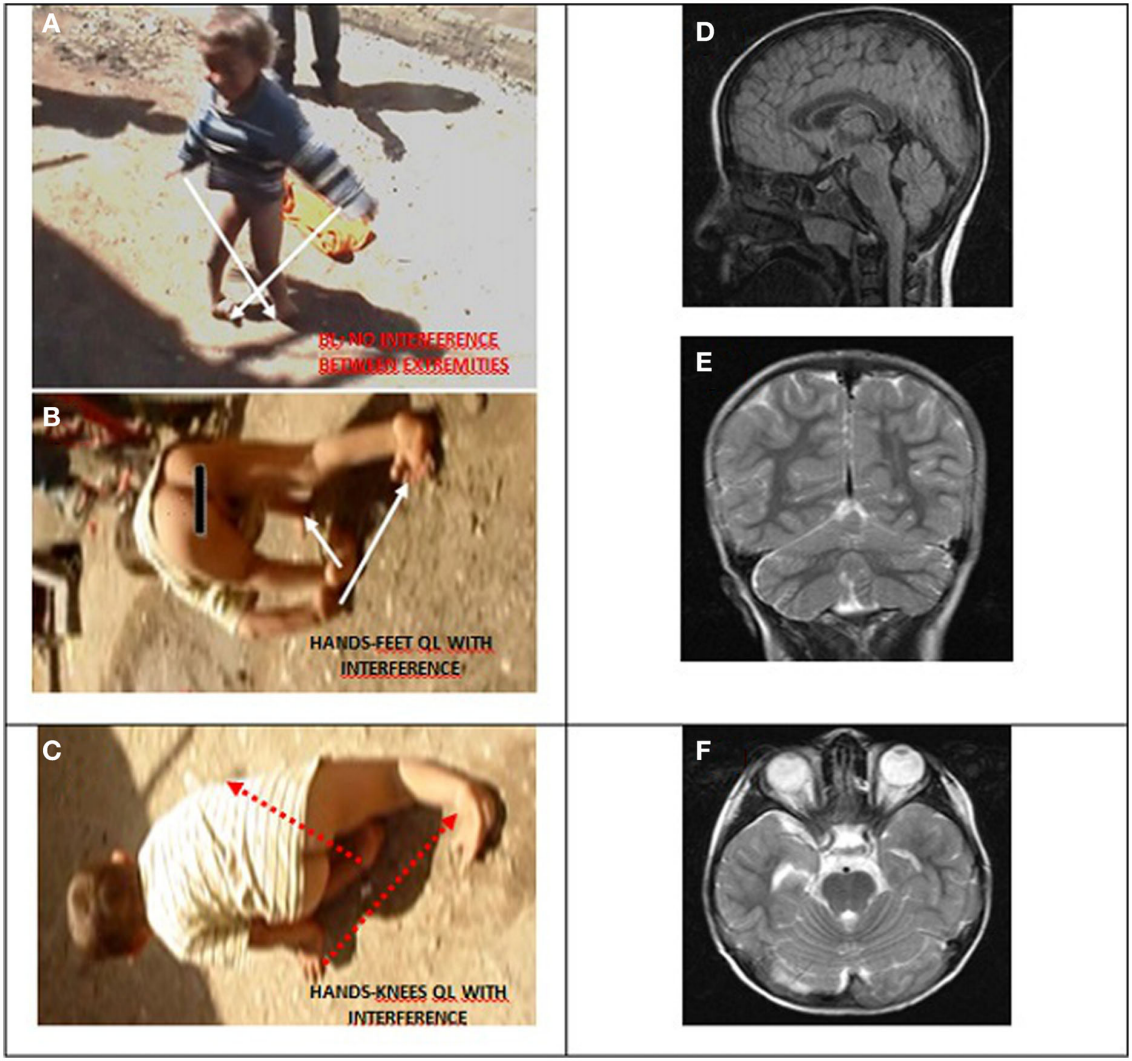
Case IV-30, 5 years old male with congenital talipes equinovarus in both feet. (A) Diagonal-sequence BL: right arm-left leg and left arm-right leg (see arrows). (B) Diagonal-sequence QL on hands and feet: left arm-right leg and right arm-left leg (see arrows). Notice the interference between the ipsilateral extremities on the left side; (C) diagonal sequence QL on hands and knees. Midsagittal (D), coronal (E), and axial (F) MRI scans.
